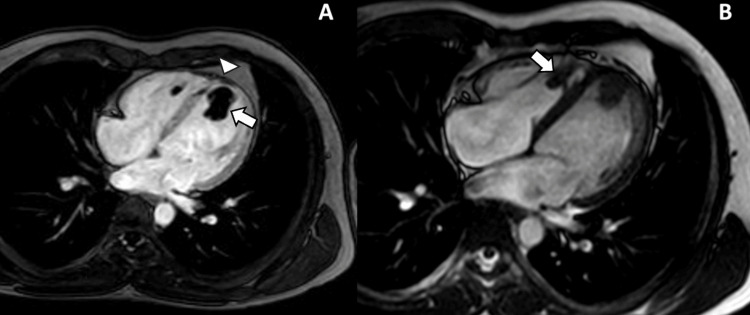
Cardiac MRI. (A) Phase-sensitive inversion recovery (PSIR) sequence demonstrates a hypointense signal at the left ventricular apex consistent with a thrombus (white arrow). The adjacent left ventricular myocardium shows region of infarction (white arrowhead). (B) A second hypointense thrombus is also visible at the right ventricular apex (white arrow).
